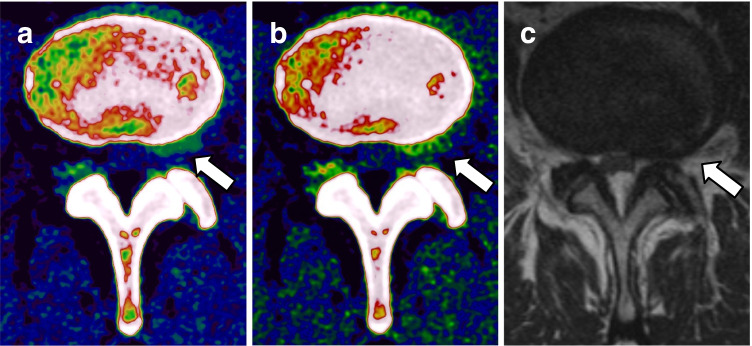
Case of a 68-year-old man suffering from lymphoma with ongoing lower back pain who underwent spine dual-energy CT imaging and MRI. Analysis of transversal color-coded VNCa images showed left-sided mediolateral lumbar disk protrusion at level L3/4 (*arrow*), which was detected on both, unenhanced (a) and contrast-enhanced portal venous phase (b) VNCa imaging by 5/5 readers. Axial T2-weighted MRI series confirmed the left-sided disk protrusion (*arrow*) (c)
